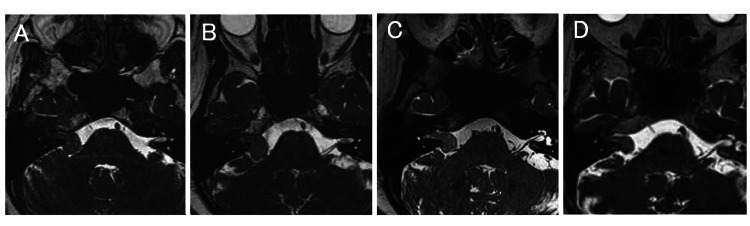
Serial constructive interference in steady state (CISS) MRI during the disease course. (A) March 18, 2013; (B) April 1, 2017; (C) March 20, 2018; (D) April 20, 2021
